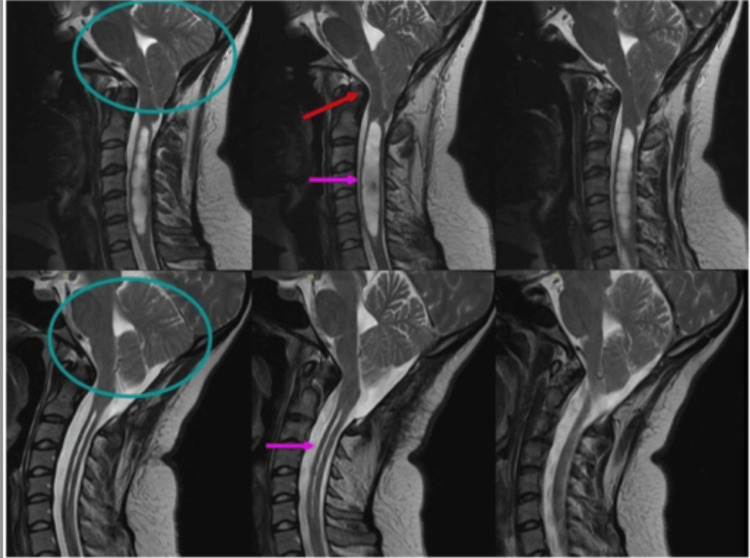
Sagittal T2-weighted MRI demonstrating CM-I before and after surgical decompression. Preoperative images show cerebellar tonsillar herniation with associated cervicomedullary compression, while postoperative images demonstrate the restoration of CSF flow and decompression at the foramen magnum Image reproduced from Open-i, National Library of Medicine (NIH) [29] (CC BY 4.0 Deed). MRI: magnetic resonance imaging; CM-I: Chiari malformation type I; CSF: cerebrospinal fluid
